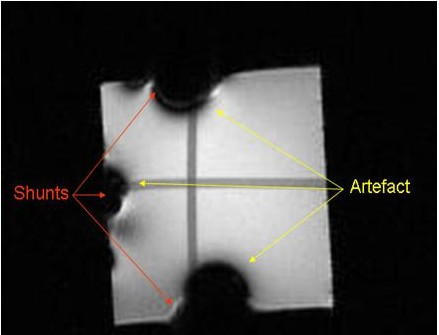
MRI scan of three shunts. The shunts were placed on testing block of glycerin (20 × 20 × 20 cm) and a 3T MRI scan was performed. Black areas show artifacts on MRI image.
